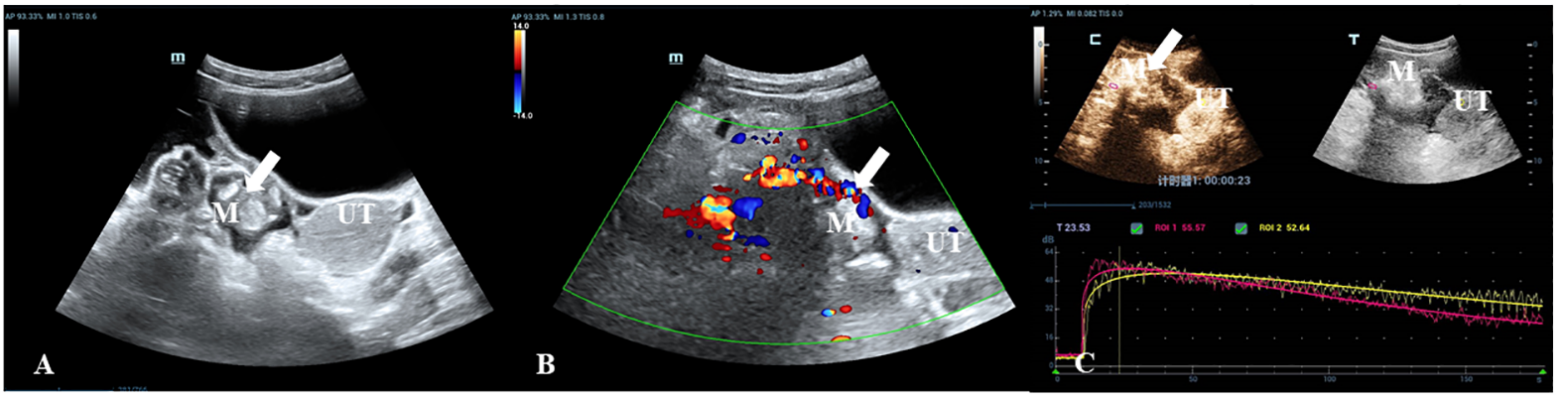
A 35-year-old woman with a pathologically proven immature teratoma. (A) Transabdominal gray-scale ultrasound shows a 30-mm complex cystic-solid mass (M) in the right adnexa. (B) Color Doppler reveals the color flow within the mass (color score=4). (C) CEU curve demonstrates that the tumor showed hyperenhancement in initial perfusion and faster washout compared with myometrium (mass: red, myometrium: yellow).
